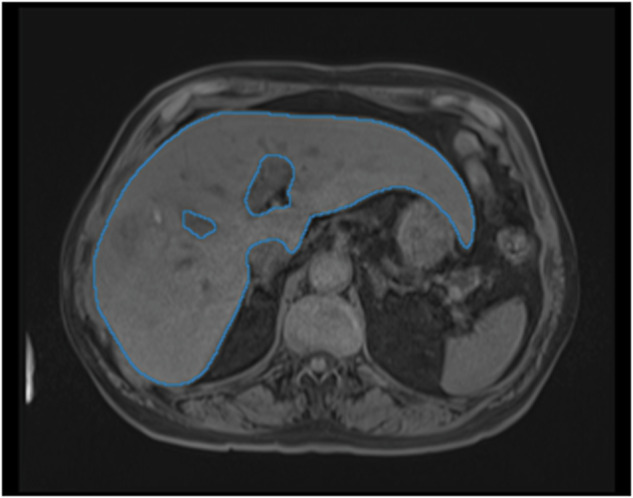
Automatic segmentation of the liver from T1 MRI scan of patient TCGA-G3-A7M7.
